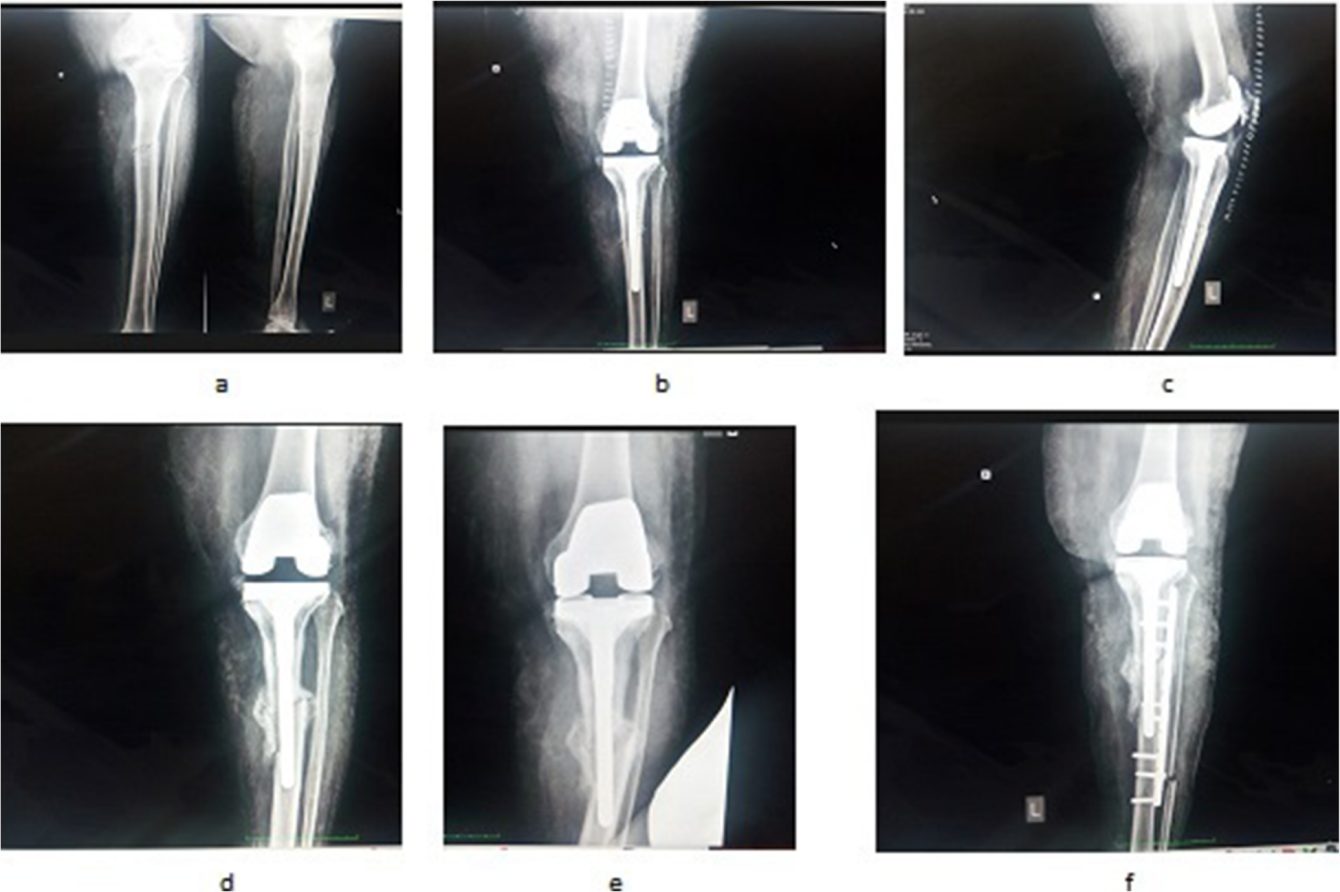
Left Knee X-rays (complication and intervention) a) Preoperative anteroposterior and lateral view showing features suggestive of osteoarthritis, with deformity with stress fracture proximal tibia; b) and c) Postoperative X-rays anteroposterior and lateral view showing correction of deformity with modular stemmed knee prosthesis with intact fibula; d) and e) Follow-up anteroposterior view showing nonunion; f) Recent follow-up anteroposterior view showing healed stress fracture with correction of deformity with modular stemmed knee prosthesis with implant (plate) in situ with resected fibula
